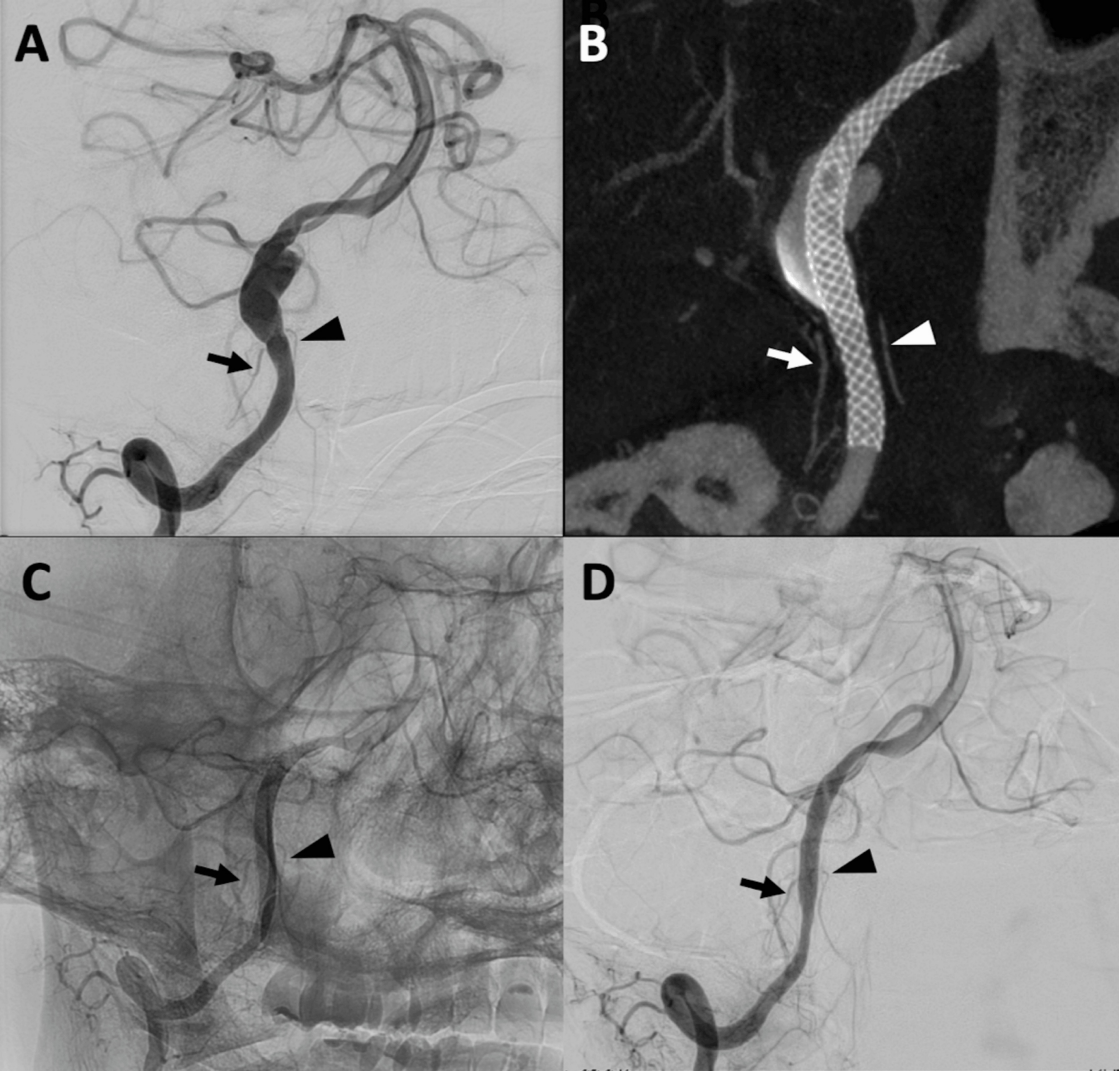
Preoperative, intraoperative, and postoperative DSA (A) Preoperative right vertebral angiogram showed the right V4 segment of VA with an irregular inner wall without stenosis, and PICA branching from the lesion (arrow) and ASA from just proximal to the lesion (arrowhead). (B) A 17% diluted contrast-enhanced 80-kV cone-beam CT immediately after the FD placement confirmed PICA (arrow) and ASA (arrowhead) patency and flow stagnation within the aneurysm and good wall apposition at PICA and ASA branches. (C) Postoperative right vertebral angiogram without subtraction on POD seven revealed complete obliteration of the aneurysm and maintained patency of PICA and ASA without in-stent stenosis. (D) Right vertebral artery angiogram at six months demonstrated complete occlusion of the VADA, while mild in-stent stenosis was noted DSA: digital subtraction angiography; VA: vertebral artery; PICA: posterior inferior cerebellar artery; FD: flow diverter; ASA: anterior spinal artery; POD: postoperative day
